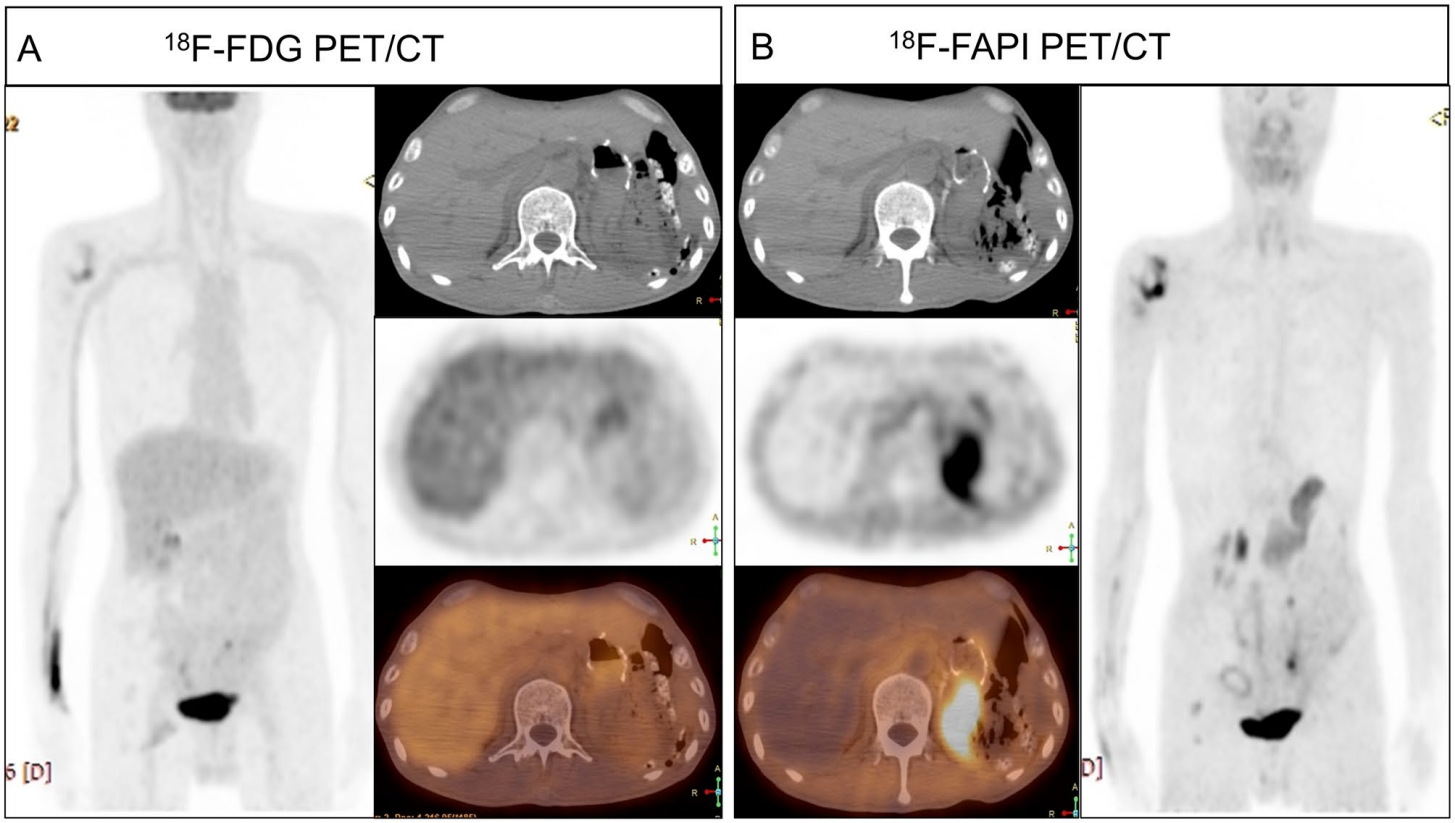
NSU in the ^18^F-FAPI after the surgery of gastrectomy and negative in the ^18^F-FDG PET/CT. A male patient, 55 years old, after surgery of gastrectomy for gastric cancer. ^18^F-FDG PET/CT (A) showed no FDG concentration in the pancreas, and ^18^F-FAPI PET/CT imaging (B) showed a high concentration of diffuse FAPI in the pancreas, with an SUVmax of 5.3. *FAPI* fibroblast activation protein, *FDG* fluorodeoxyglucose, *PET/CT* positron emission tomography/computed tomography. *SUVmax* maximum standardized uptake value.
